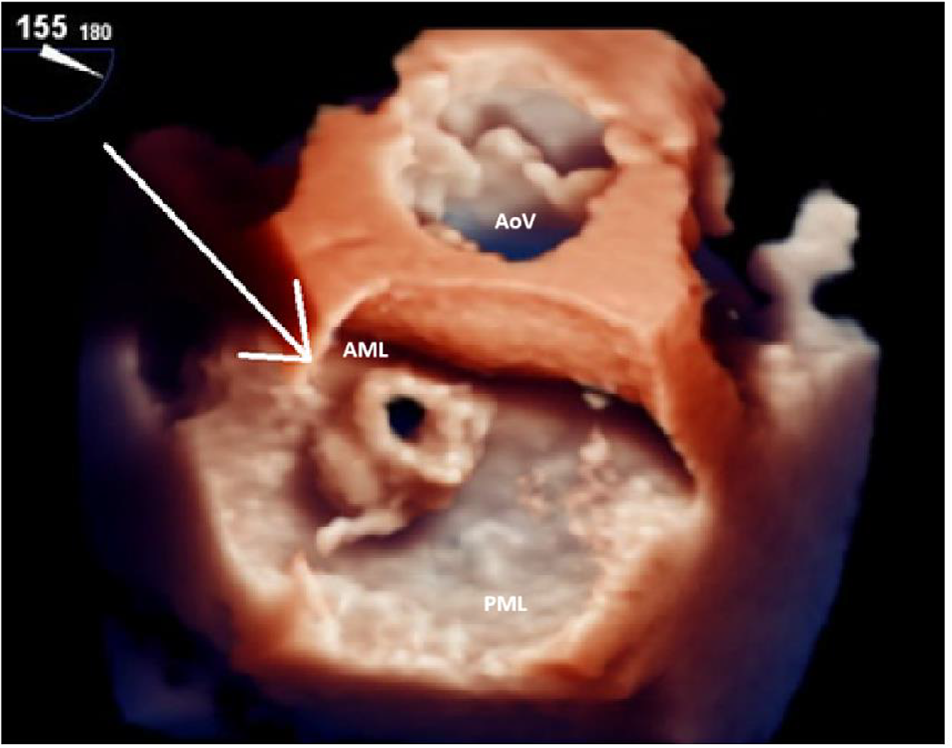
Three‐dimensional transesophageal echocardiogram demonstrating a large, irregular vegetation (white arrow) attached to the mitral valve with associated perforation of the anterior mitral leaflet. AML, anterior mitral leaflet; AoV, aortic valve (opened); PML, posterior mitral leaflet (closed).
